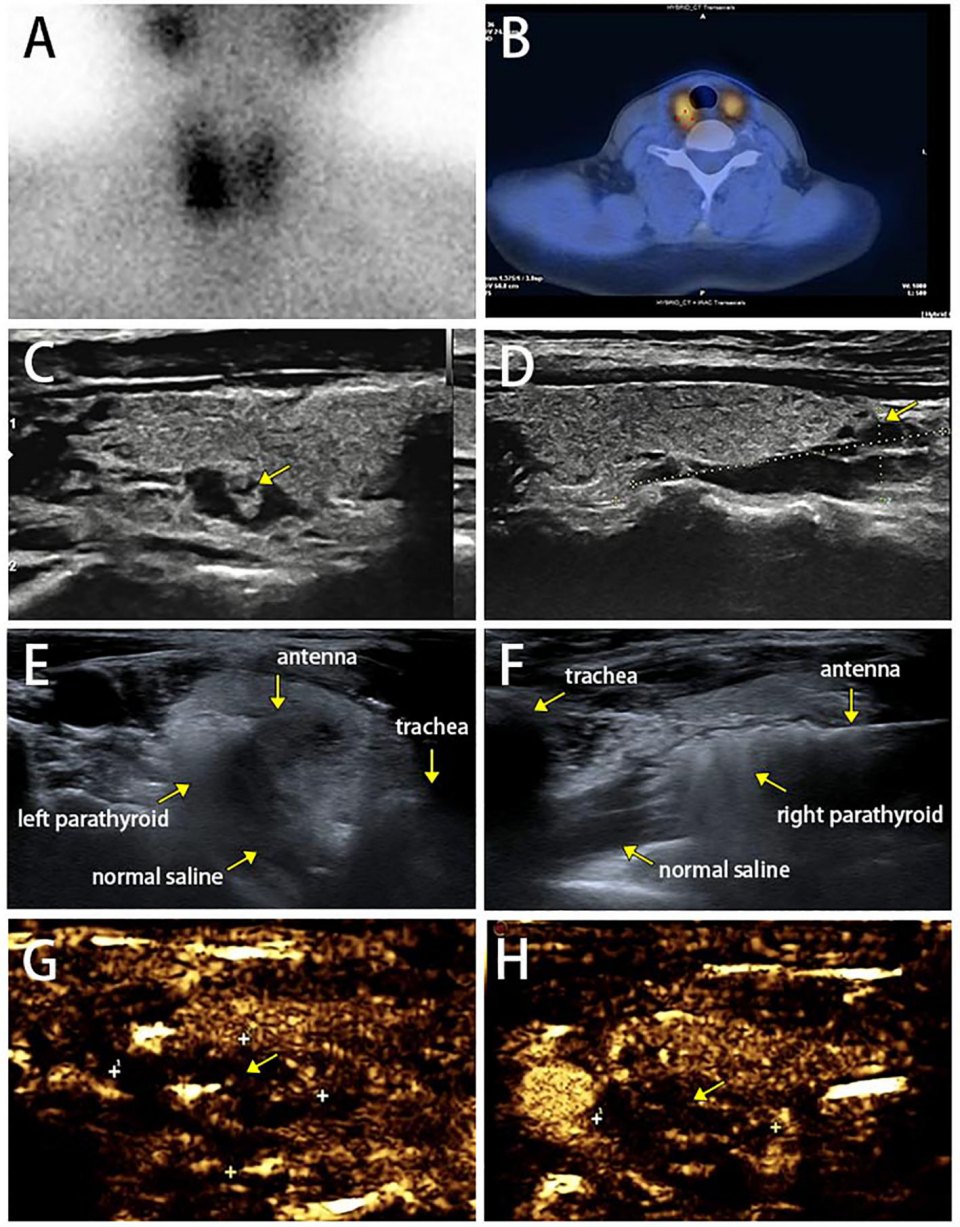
(A, B) Dual-phase 99mTc-MIBI parathyroid scan on parathyroid glands with three focal uptakes. (C, D) Ultrasound scan of parathyroid glands. (E, F) Complete hyperechoic area in the parathyroid glands indicated a complete ablation. (G ,H) Postoperative contrast enhanced ultrasound for parathyroid glands did not show blood flow in the remaining lesions.
